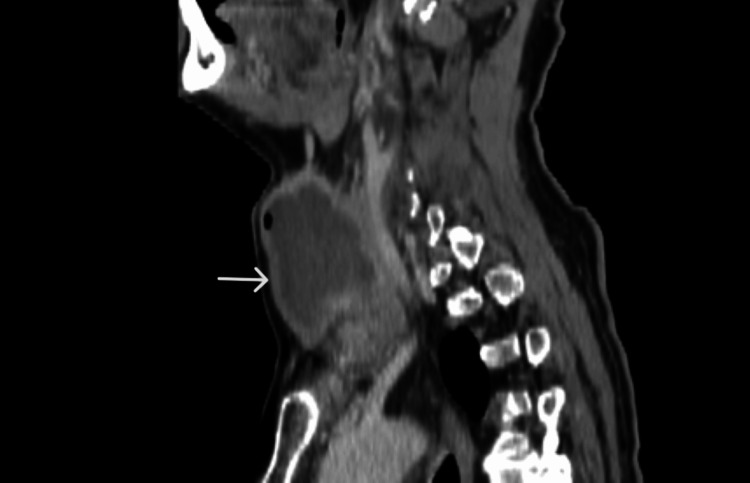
Neck CT - sagittal view. Cystic degeneration is prominent (white arrow).
